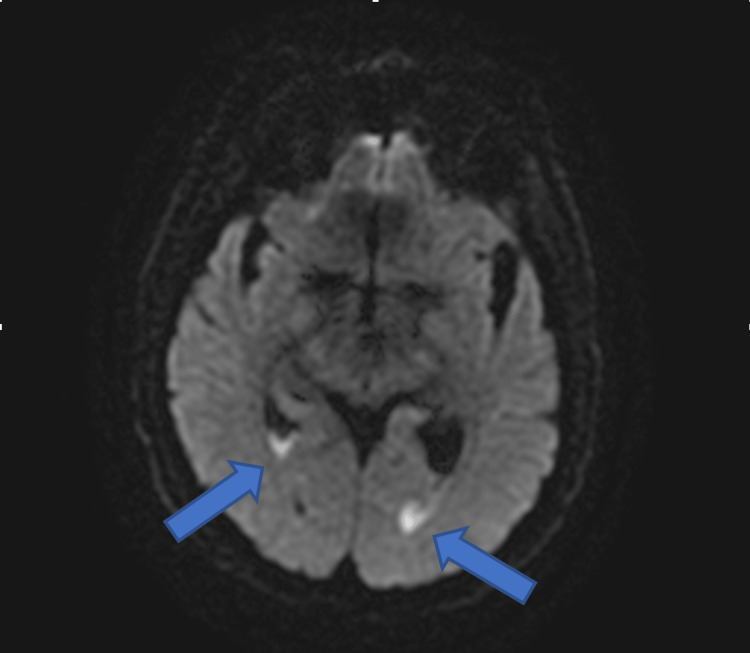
Diffusion-weighted imaging (DWI) demonstrating layering purulent debris within the bilateral occipital horns (blue arrows).
